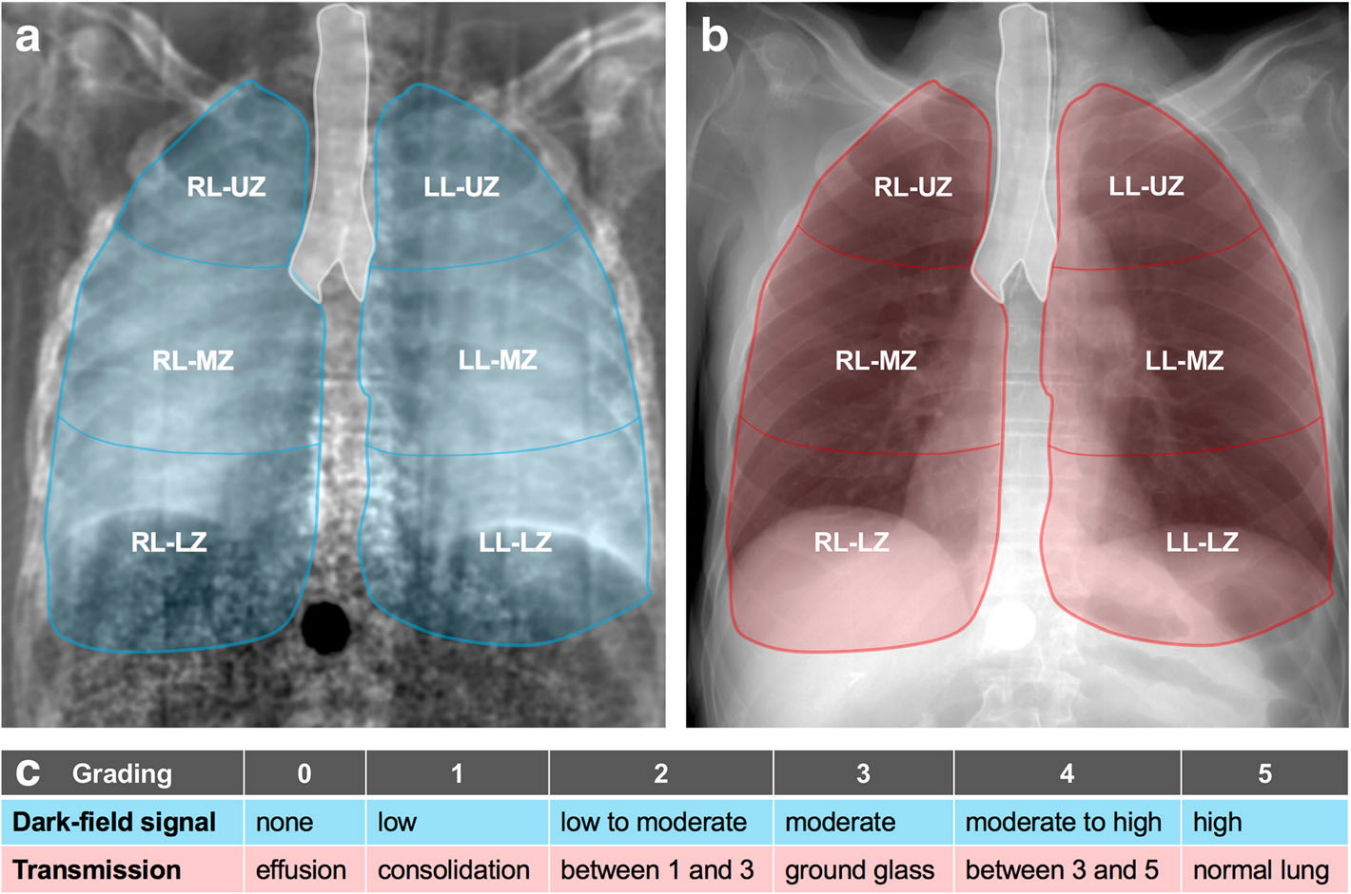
Visual evaluation scheme and grading scale for dark-field chest radiographs and conventional x-rays. In dark-field (a) and conventional (b) chest x-ray, lungs are divided into six regions: right lung-upper zone (RL-UZ), right lung-middle zone (RL-MZ), right lung-lower zone (RL-LZ), left lung-upper zone (LL-UZ), left lung-middle zone (LL-MZ), and left lung-lower zone (LL-LZ). In each region, dark-field (a) and transmission (b) signals are visually graded using a (c) 6-point ordinate scale
